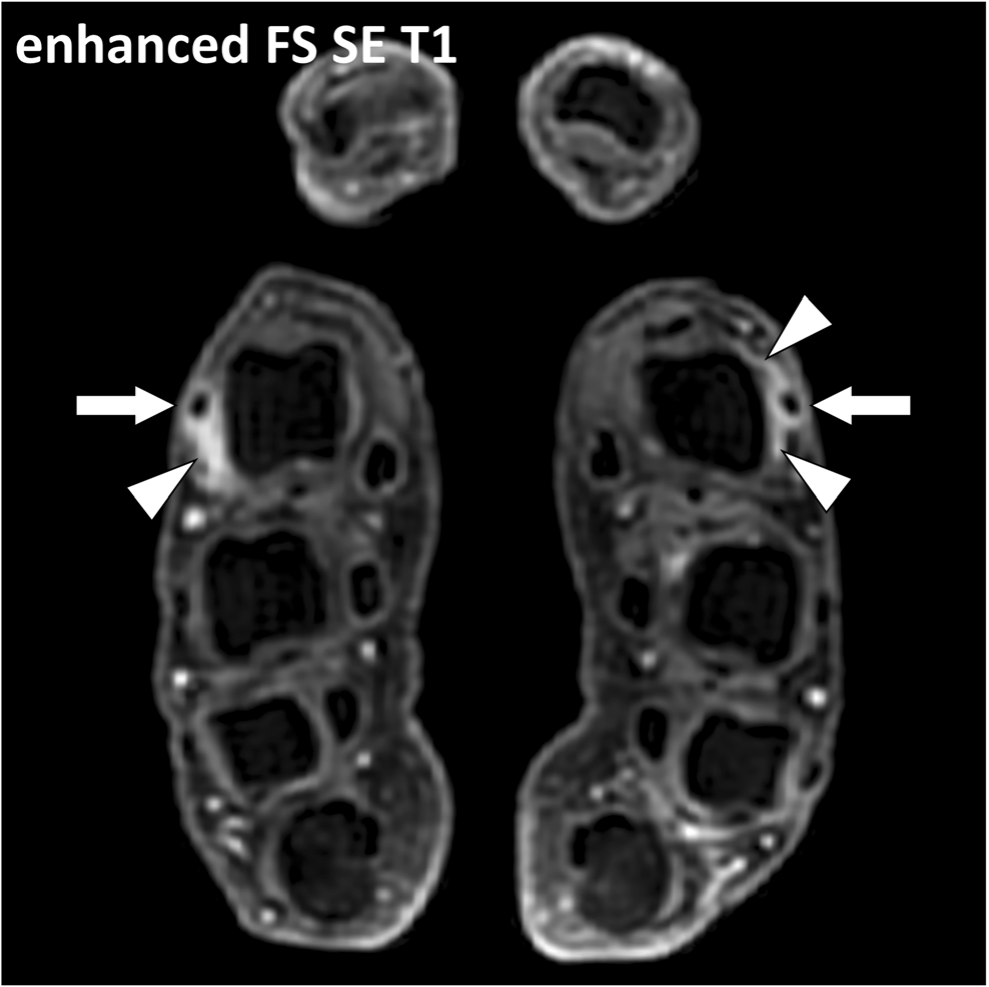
Contrast-enhanced fat-suppressed T1-weighted axial image of the hands of a 29-year-old female with seropositive rheumatoid arthritis. Peritendinitis of the extensor digitorum tendons of the second digits (arrows) is associated with thickening and enhancement of the connective tissue around the tendons and the dorsal parts of the sagittal bands (arrowheads). The term “peritendinitis” seems more appropriate than “tenosynovitis” as there is no synovial sheath around the extensor tendons at the level of the metacarpophalangeal joints
